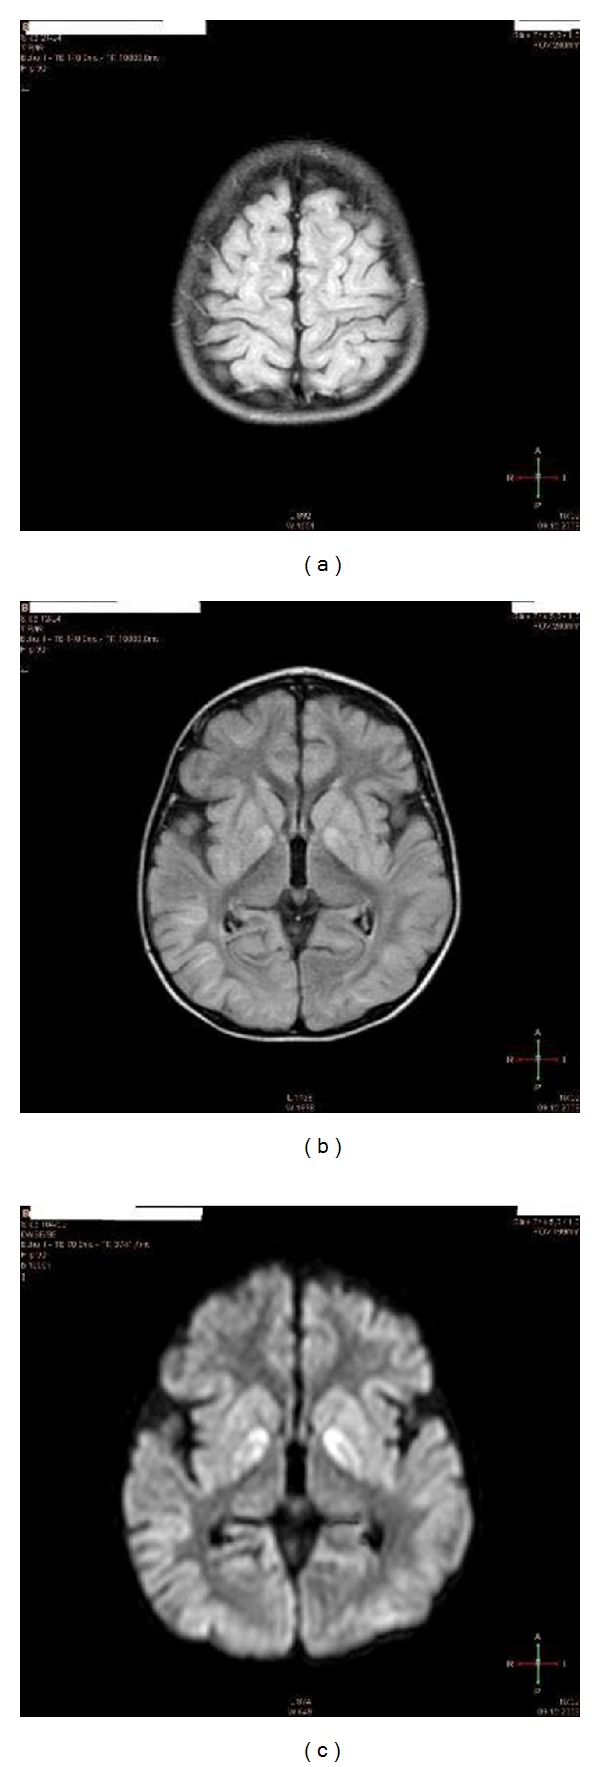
Brain MRI of the patient (L. B.) 2 weeks after cardiac arrest. Note, signs of severe global ischemia in cortical structures as evidenced by (a) signal hyperintensity of gyri in almost entire cortex (FLAIR sequences) and basal ganglia (b), including caudate nucleus and putamen (c) (FLAIR DWI sequences with contrast media) (see video S1, S2 in Supplementary Material [44]).
